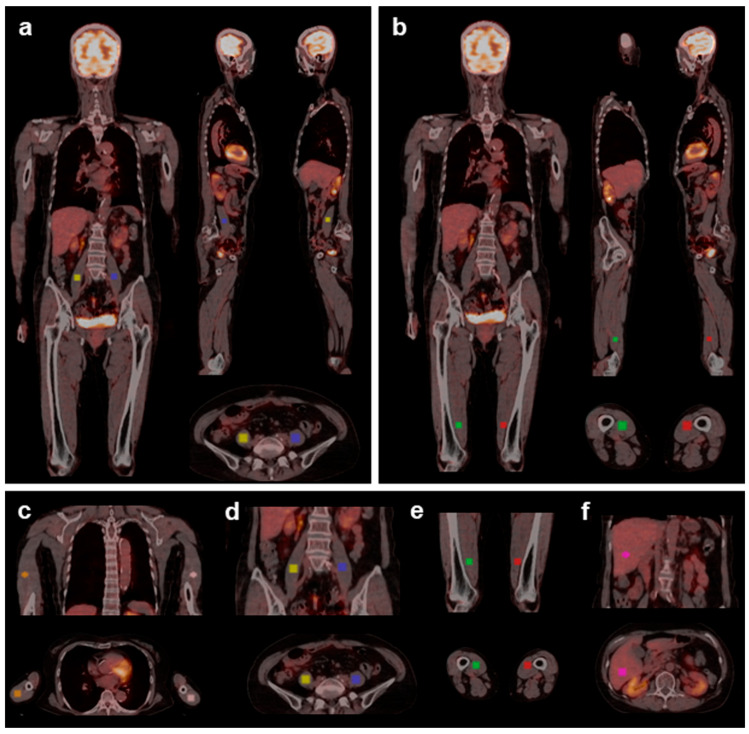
Representative whole-body FDG PET/CT images of ROI placement on the psoas (a) and vastus medialis (b). Three-dimensional ROIs (5.1 cm^3^) are placed at the widest and thickest part of each muscle belly by confirming the coronal, sagittal, and cross-sectional images. ROI placement on the skeletal muscles and liver: The major gait-related muscles ((c), triceps; (d), psoas; (e), vastus medialis) are identified bilaterally on PET/CT fusion images. The liver ROI is used as the standard reference for glucose uptake (f). The upper and lower rows represent coronal and cross-sectional images, respectively. ROI: region of interest.
